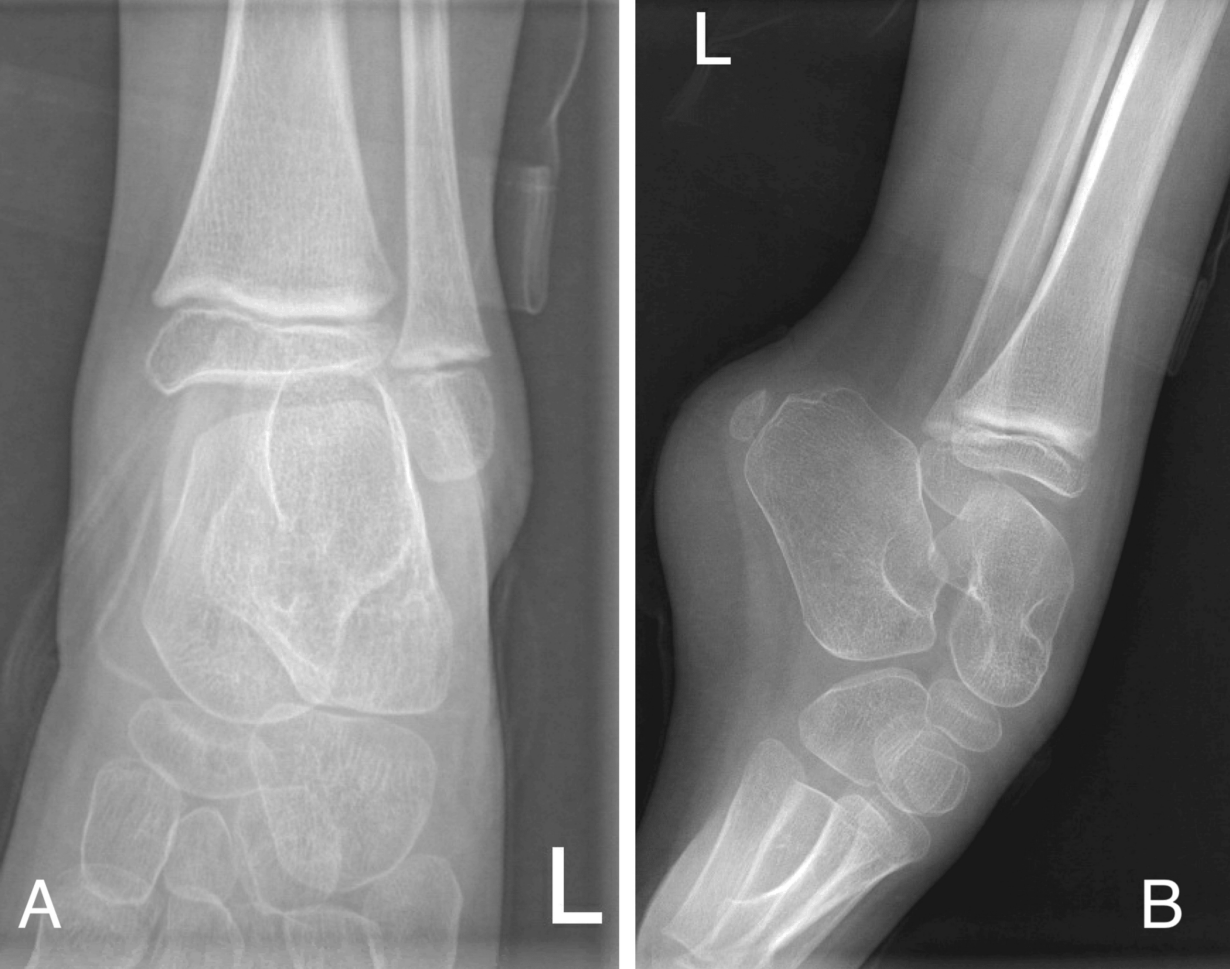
Preoperative X-ray of the left side Preoperative anterior-posterior (A) and lateral (B) X-ray of left ankle showing equinus deformity without obvious bony abnormality.
